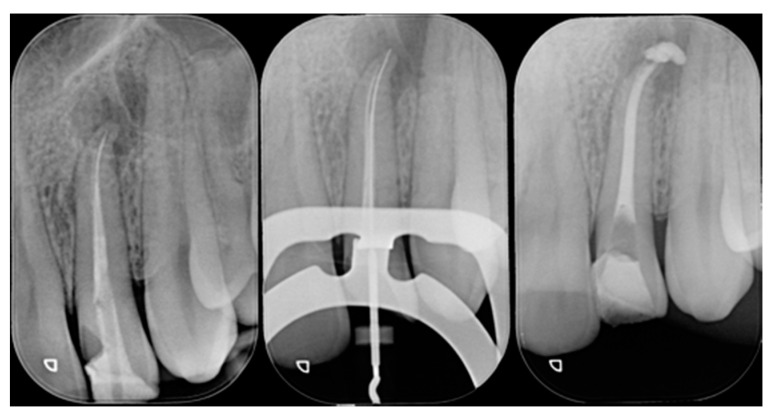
Initial x-ray, during and after endodontic retreatment. Reprinted from Restauri diretti nei settori anteriori, G. Paolone, S. Scolavino, © 2021, with permission from Quintessence Publishing Italy.
